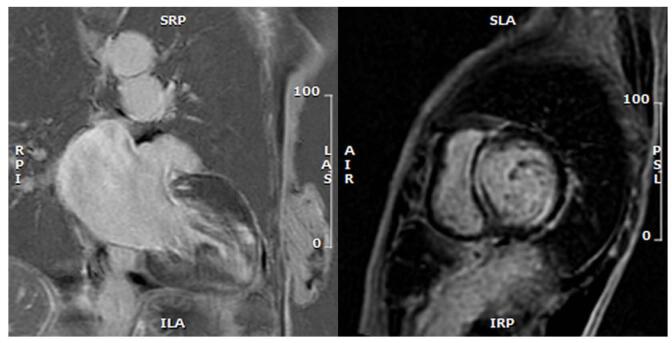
Cardiac MRI (Patient#3): Diffuse sub-endocardial LGE with basal predominance.
